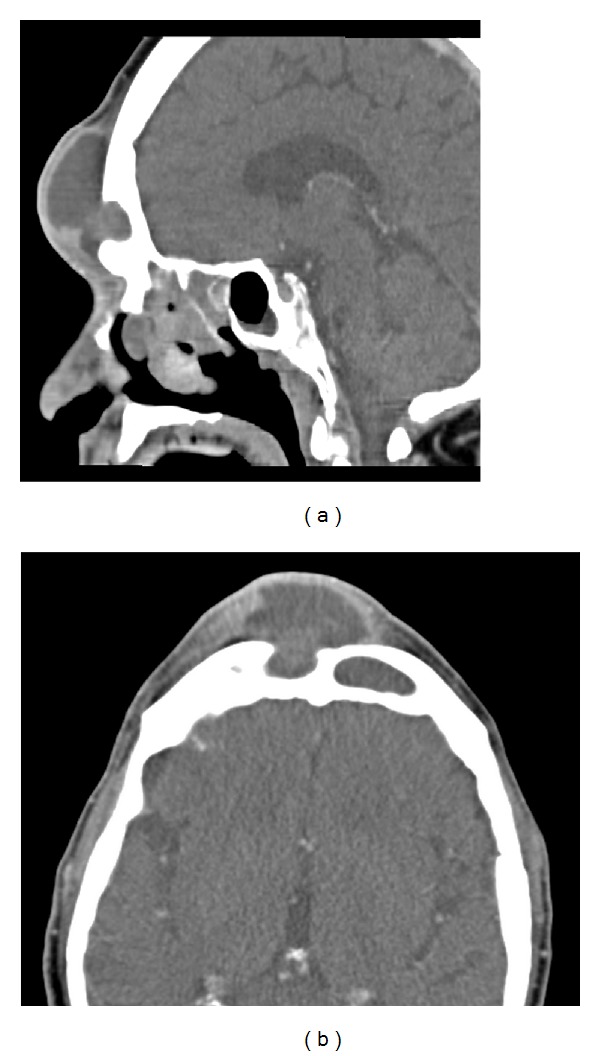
Osteoplastic frontal sinus obliteration (OSFO) in the setting of anterior table erosion. Sagittal (a) and axial (b) CT scan of sixty seven year old man who presented with pansinusitis and right frontal mucopyocele and resulting anterior table erosion. After urgent decompression and six week course of intravenous antibiotics, this patient underwent successful OFSO and has remained symptom-free for eighteen months.
